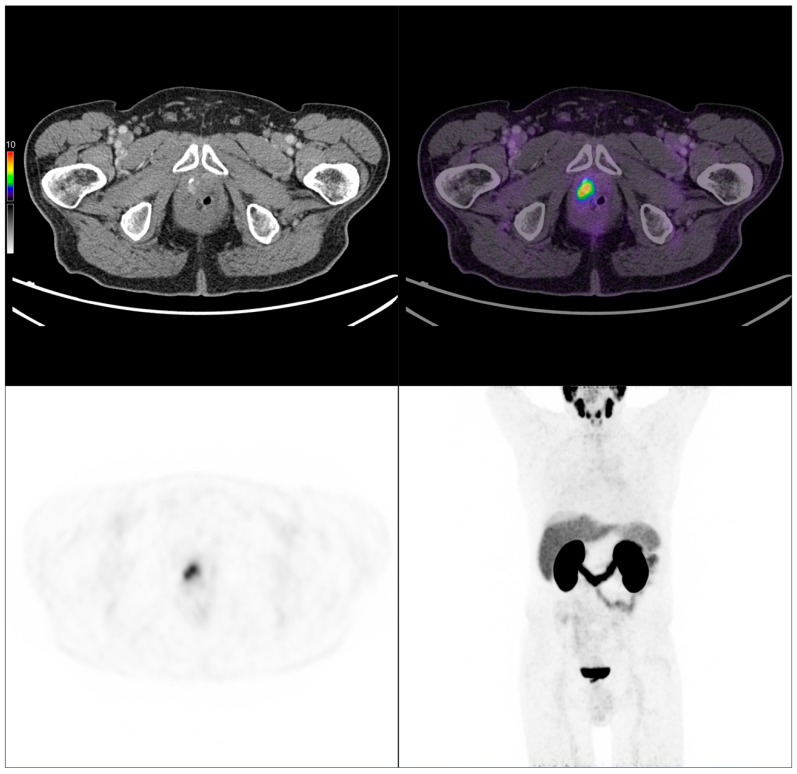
PSMA PET/CT scan of an 84-year-old patient with biochemical recurrence (PSA: 0.67 ng/mL). Initially, the Gleason score was 4 + 3 = 7, pT3 pN0 M0, and treated with radical prostatectomy. Imaging performed following intravenous administration of 141 MBq of [^68^Ga]Ga-PSMA-11 revealed a local recurrence.
